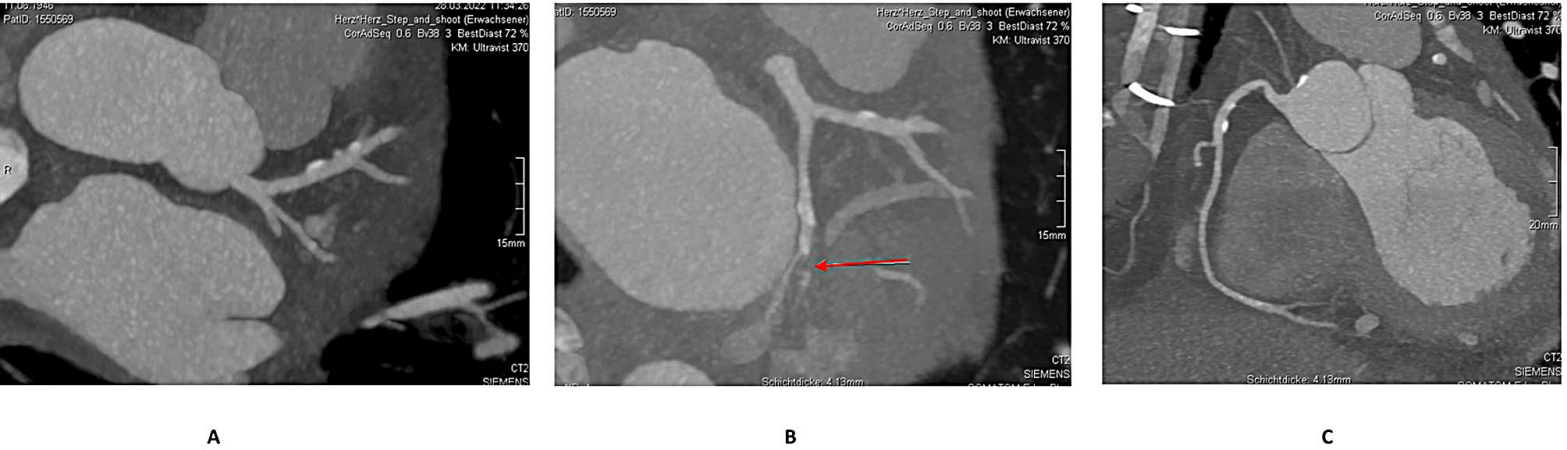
Preoperative screening for coronary disease in patient II Coronary thoracic CT-angiogram reconstruction of patient II reveals an intact main left coronary artery (Fig. 3A), suspicion (red arrow) of an occluded first marginal branch (Fig. 3B), and an intact right dominat coronary artery (Fig. 4C)
